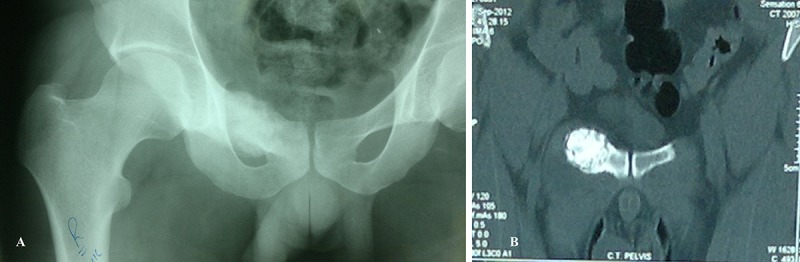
A) Radiograph showing cartilaginous growth in right superior ramus of Pubic Bone, suggestive of osteochondroma. B) Noncontrast computed tomography of pelvis showing exostosis of 3×2.5 cm involving the right superior pubic ramus.
